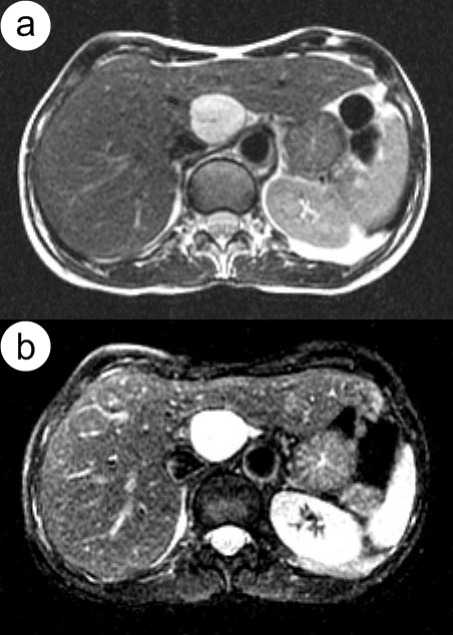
Hepatic hemangioma. Moderately (a) and heavily (b) T2-weighted MR images show typical bright lesions.
